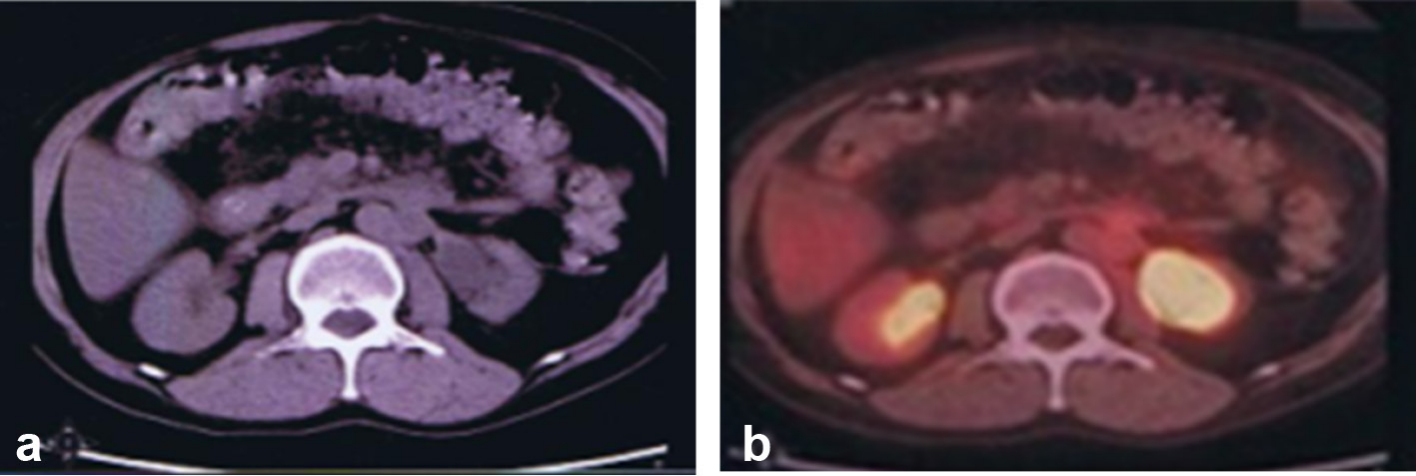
Contrast-enhanced CT abdomen (a) and CT-positron emission tomography scan
